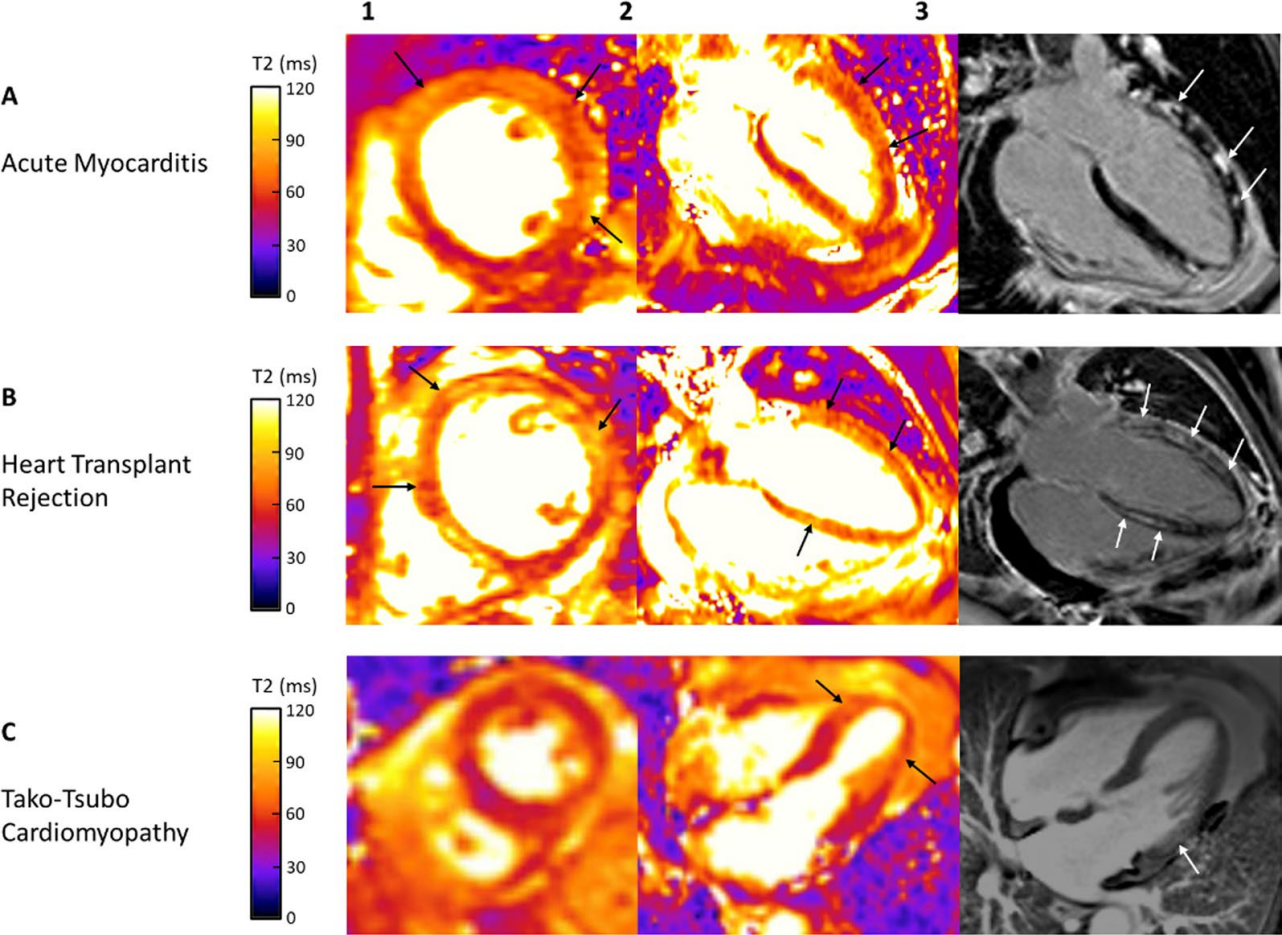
Cardiovascular Magnetic Resonance Imaging in Patients with Presence of Myocardial Inflammation. Panel 1–2: T2 mapping; Panel 3: late gadolinium enhancement imaging. Panel 1—midmyocardial short axis view; Panel 2, 3—horizontal long axis view. A Diffuse myocardial edema/inflammation (Panel 1–2; black arrows) with corresponding prominent midmyocardial to subepicardial nonischemic enhancement (Panel 3; white arrows) most prominent in the lateral wall in a patient with myocarditis. B Patient post heart transplant with diffuse myocardial edema/inflammation (Panel 1–2; black arrows) with striking diffuse linear nonischemic midmyocardial enhancement most prominent in the septal and lateral walls (Panel 3; white arrows). Findings concerning for heart transplant rejection. C: Patient with Takotsubo cardiomyopathy with myocardial edema/inflammation in the mid to apical segments (Panel 1–2; black arrows) with lack of corresponding enhancement in the mid to apical segments. Mild patchy midmyocardial nonischemic fibrosis in the basal lateral wall (Panel 3; white arrow). Panel A, B—T2 prepared (rectangular preparation) pulse sequence with balanced steady state free precession (bSSFP) readout utilized to acquire T2 maps during diastole (1.5 T MAGNETOM Avanto, Siemens Healthineers). Panel C—T2-prepared bSSFP pulse sequence with the use of rectangular T2 preparation pulse utilized to acquire T2 maps during diastole (1.5 T MAGNETOM Sola, Siemens Healthineers)
